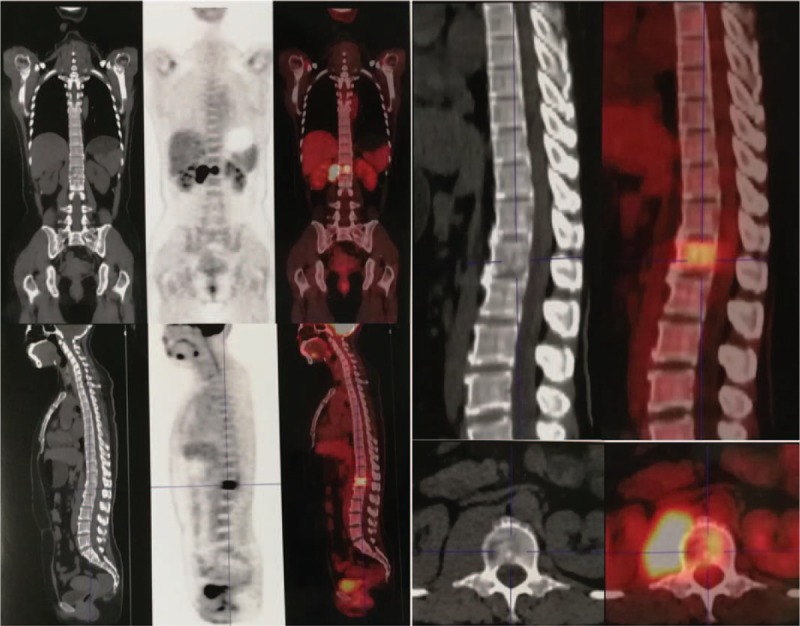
Positron emission tomography-computed tomography revealed malignant tumors of the L1 with paravertebral involvement.
